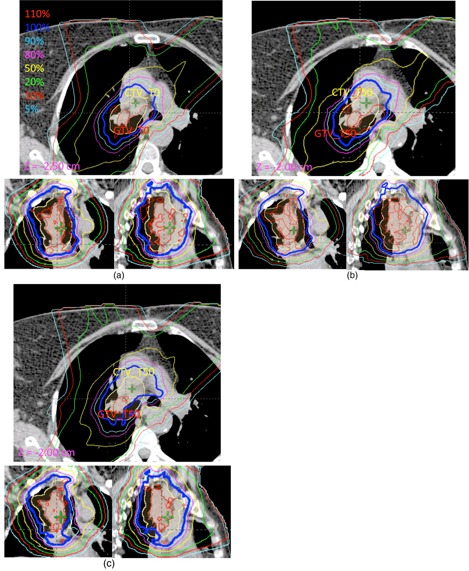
CT images showing dose deformation and 4D dose calculation for (a) one slice of a predeformed T0 dose at T0 of the 4D CT (Z = ‐2.5 cm), (b) same slice of postdeformed T0 dose at T50 of the CT (Z = ‐2.0 cm), and (c) same slice of T50 dose at T50 of the CT (Z = ‐2.0 cm).
